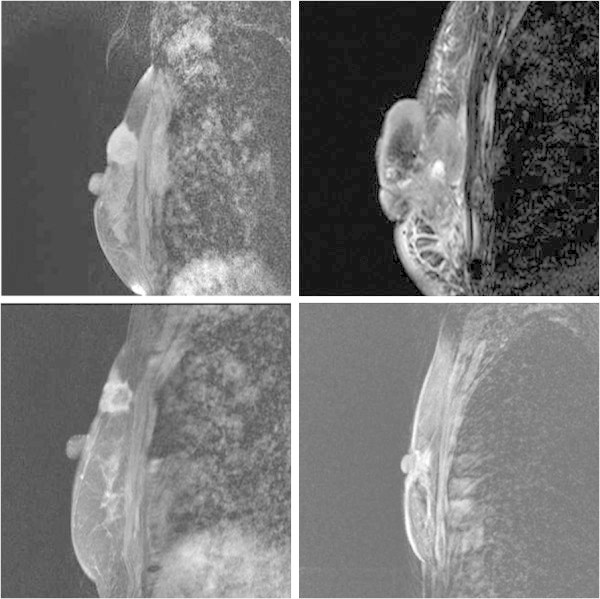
MRI findings of the bilateral breast cancers at pre-and post-treatment. MRI showed a distinctly round contrasting mass of 27 × 19 mm in the right breast (upper right) and a distinctly irregular contrasting mass of 57 × 42 mm in the left breast (upper left) at pre-treatment. Each mass had clearly skin invasion, by contrast each had not reached to the chest wall. After the treatment the left tumor showed a significant tumor reduction (lower left), but not right tumor (lower right).
